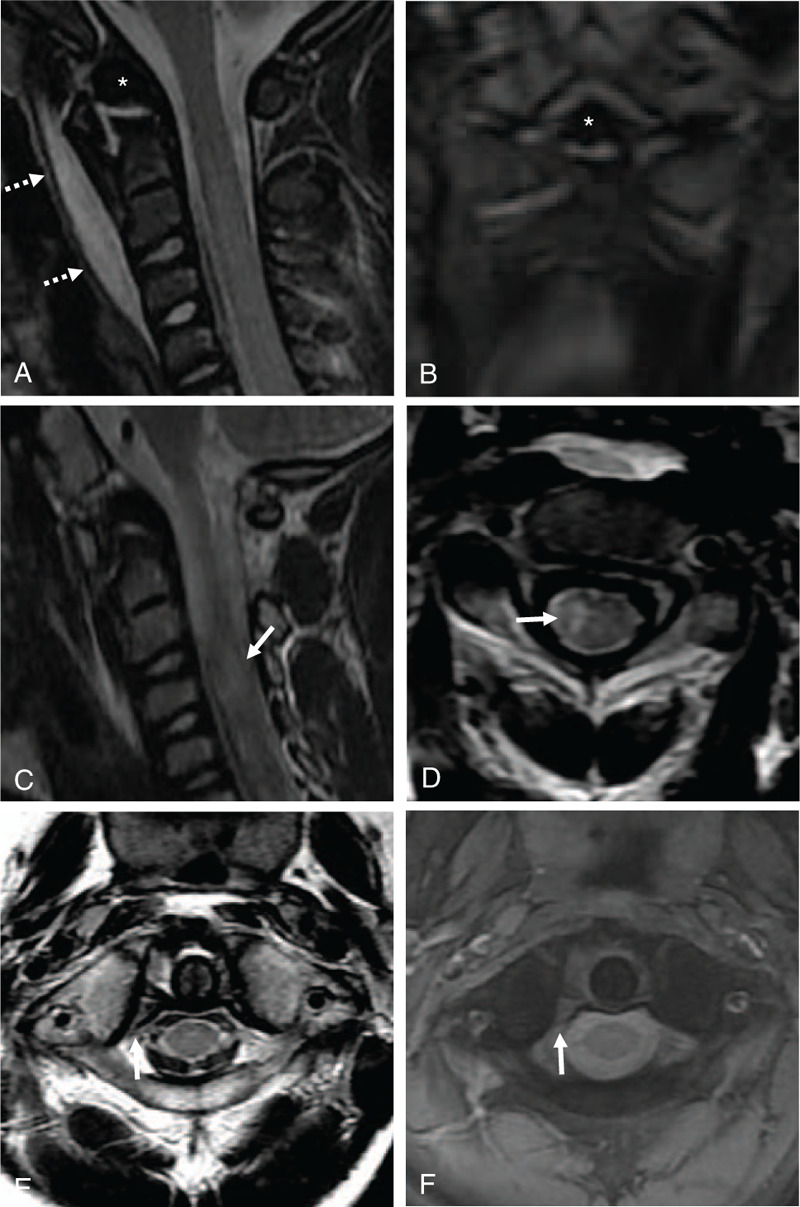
Midline sagittal and coronal (A and B) magnetic resonance imaging (MRI) showing retropharyngeal hematoma (dotted white arrows) and transverse dens fracture (white asterisks). Right parasagittal and axial T2-weighted (C and D) MRI showing intramedullary hemorrhage that occupied about 35% of the right side of C3–4 spinal cord (white arrows). Axial T2- and T1-weighted (E and F) MRI showing injury of transverse atlantal ligament (TAL) (white arrows) on right side of lateral mass of C1, which was type 1B TAL injury (according to Dickman classification).
